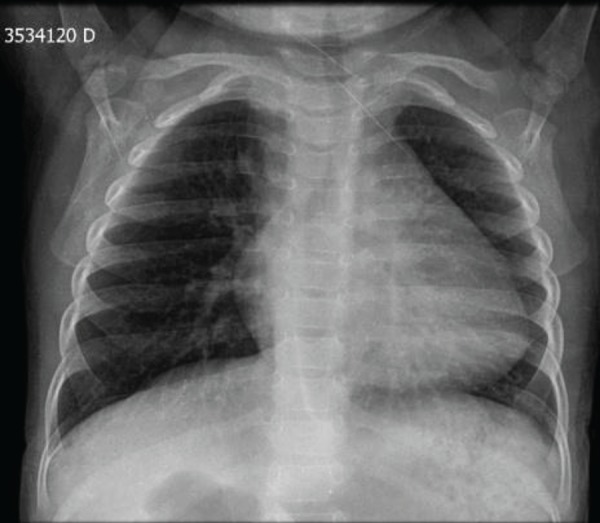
Chest radiograph showed increased cardiac area and normal pulmonary
